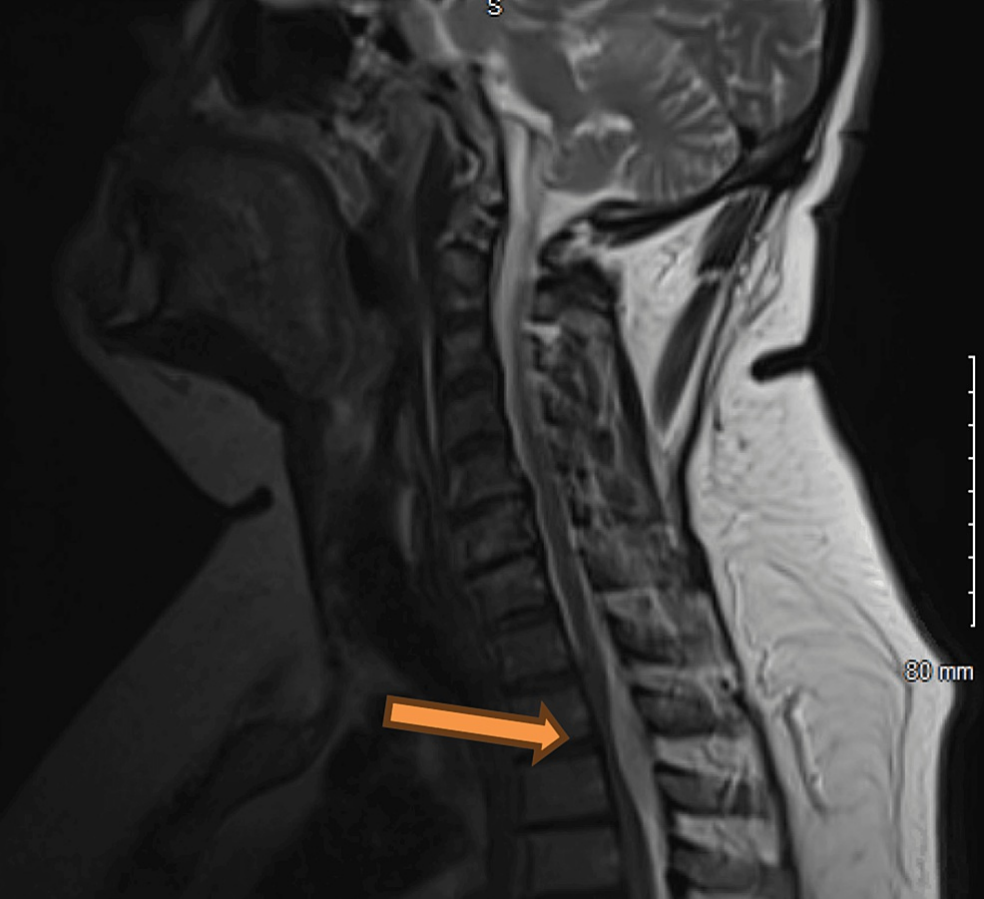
T2-weighted image showing the mass.
